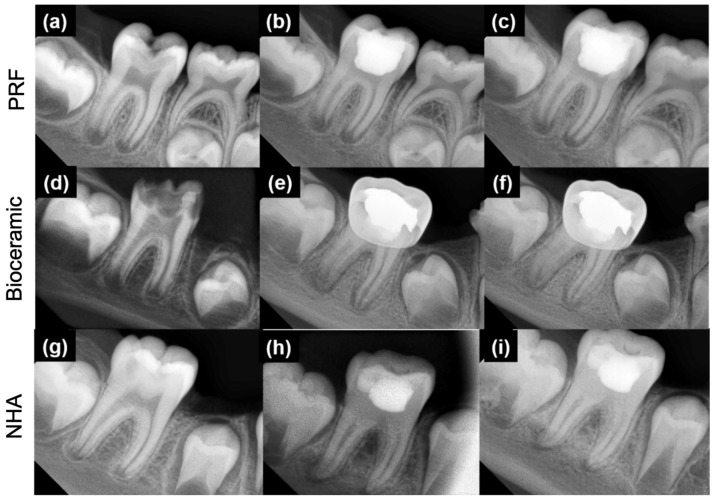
(a) Preoperative radiograph of an immature mandibular molar; (b) no complete apical closure at 6 months; (c) no complete apical closure at 12 months “continued apical closure”; (d) preoperative radiograph of an immature mandibular molar; (e) no complete apical closure at 6 months “continued apical closure”; (f) complete apical closure at 12 months; (g) preoperative radiograph of an immature mandibular molar; (h) complete apical closure at 6 months; (i) complete apical closure at 12 months.
